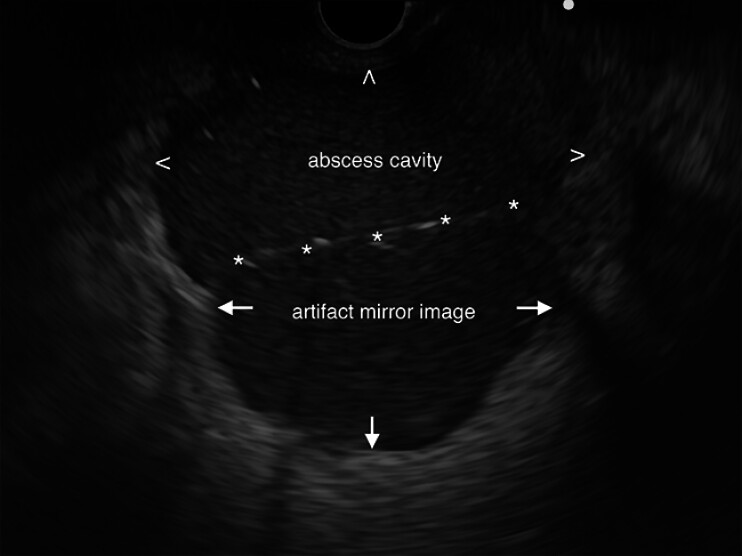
Endoscopic ultrasound image of the abscess (arrowheads), with mirror-image artifact (arrows) arising at the highly reflective interface between gas and fluid in the abscess cavity (asterisks).
